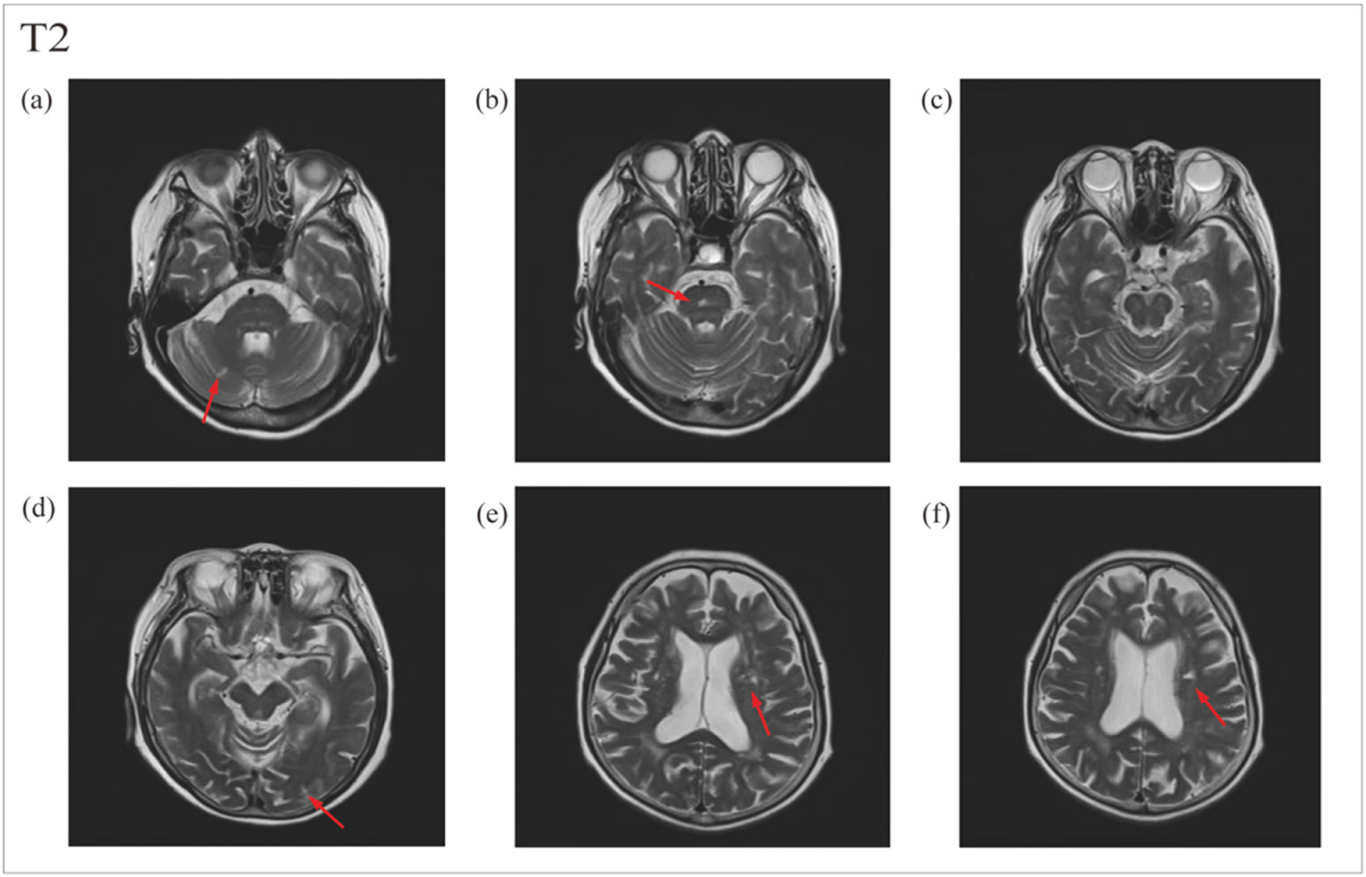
T2 sequence of cerebral MRI. (a–f) Multiple lacunar infarcts in cerebral cortex, subcortex and cerebellum.
